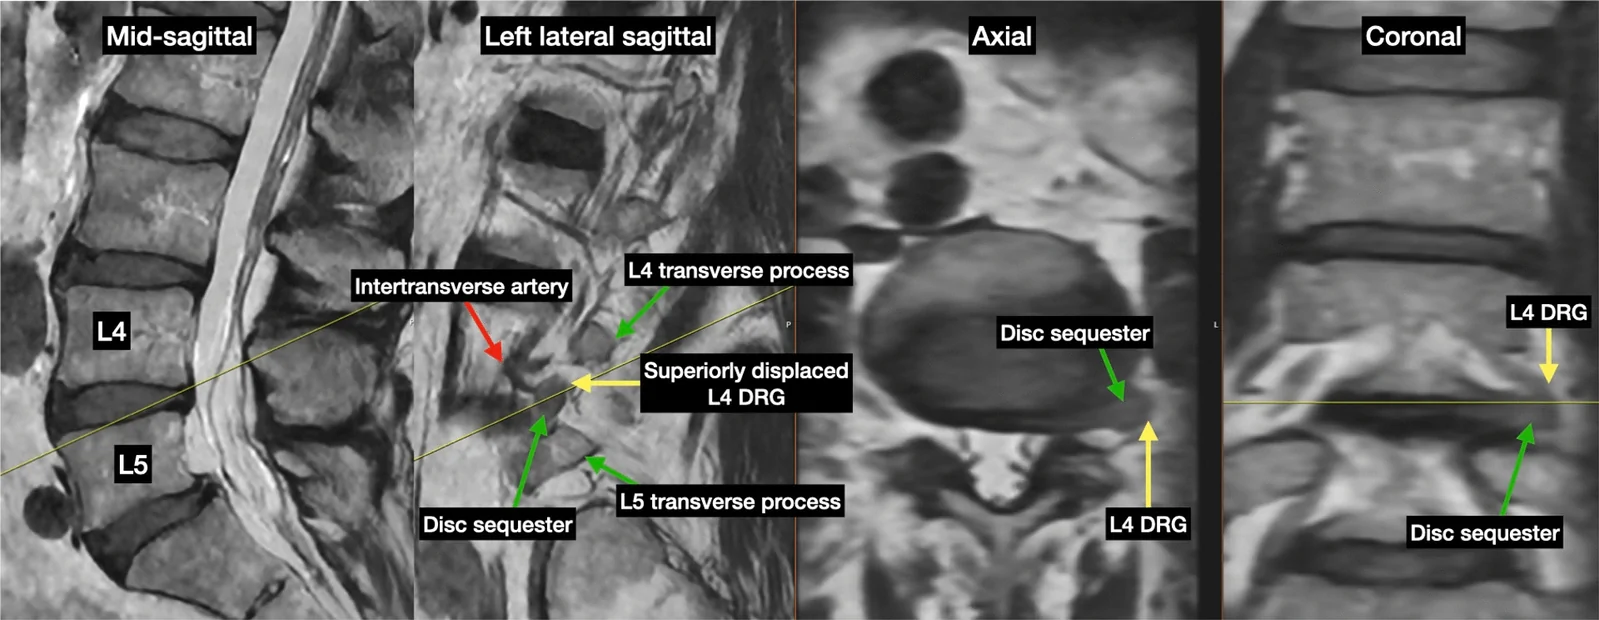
T2-weighted MR imaging showing a left-sided extraforaminal disc herniation at L4-L5 that lifts the dorsal root ganglion of the L4 nerve upwards, with coronal, axial, left lateral sagittal, and mid-sagittal views. Note the close relationship of the disc sequester and superiorly displaced L4 dorsal root ganglion with the intertransverse artery, arising from the lumbar segmental artery
